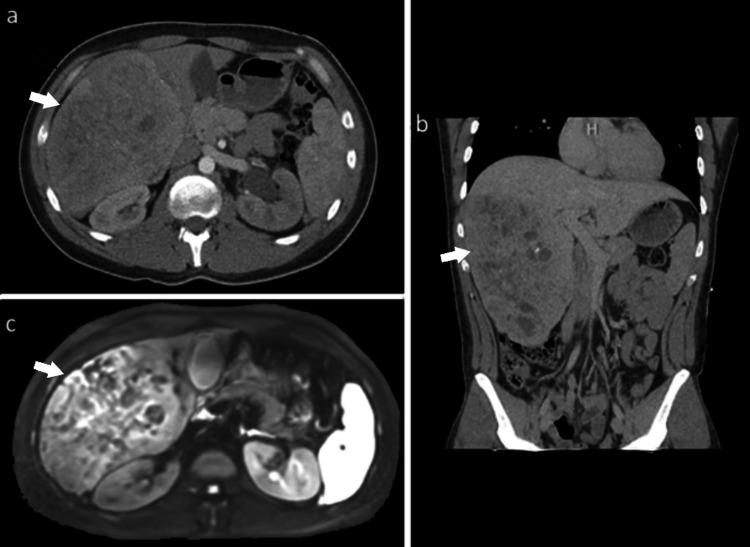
Pre-operative images a) Contrast CT - transverse, b) Contrast CT - coronal, c) T2-weighted transverse MRI. Subsequent imaging was obtained after a large right hepatic mass was discovered incidentally on CT evaluation for nephrolithiasis. Arrows point towards the right hepatic mass.
